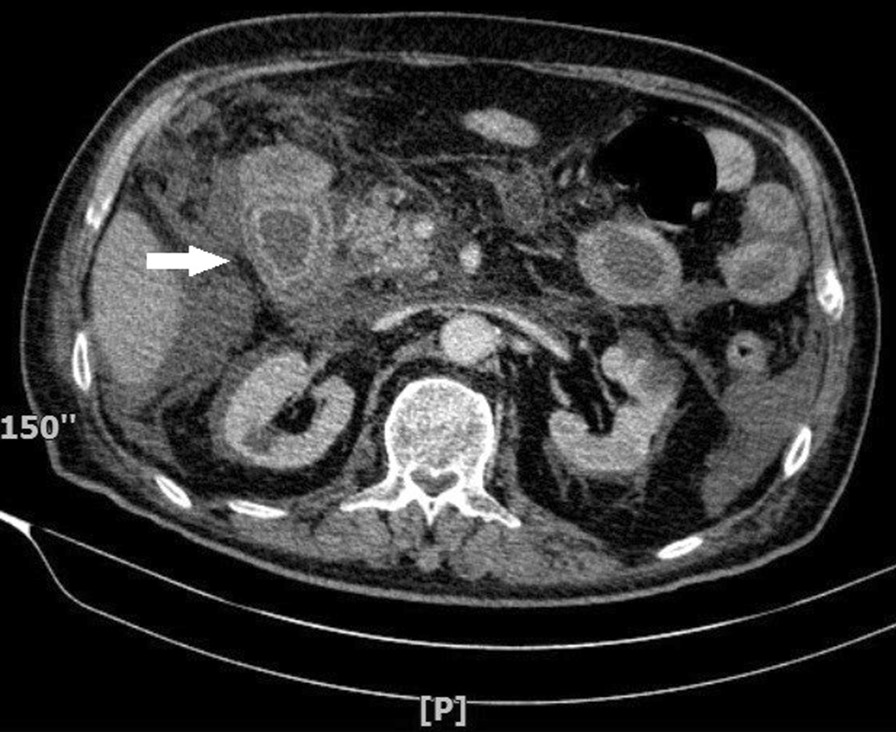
Contrast-enhanced CT scan in favor of ischemic duodenum (white arrow)
